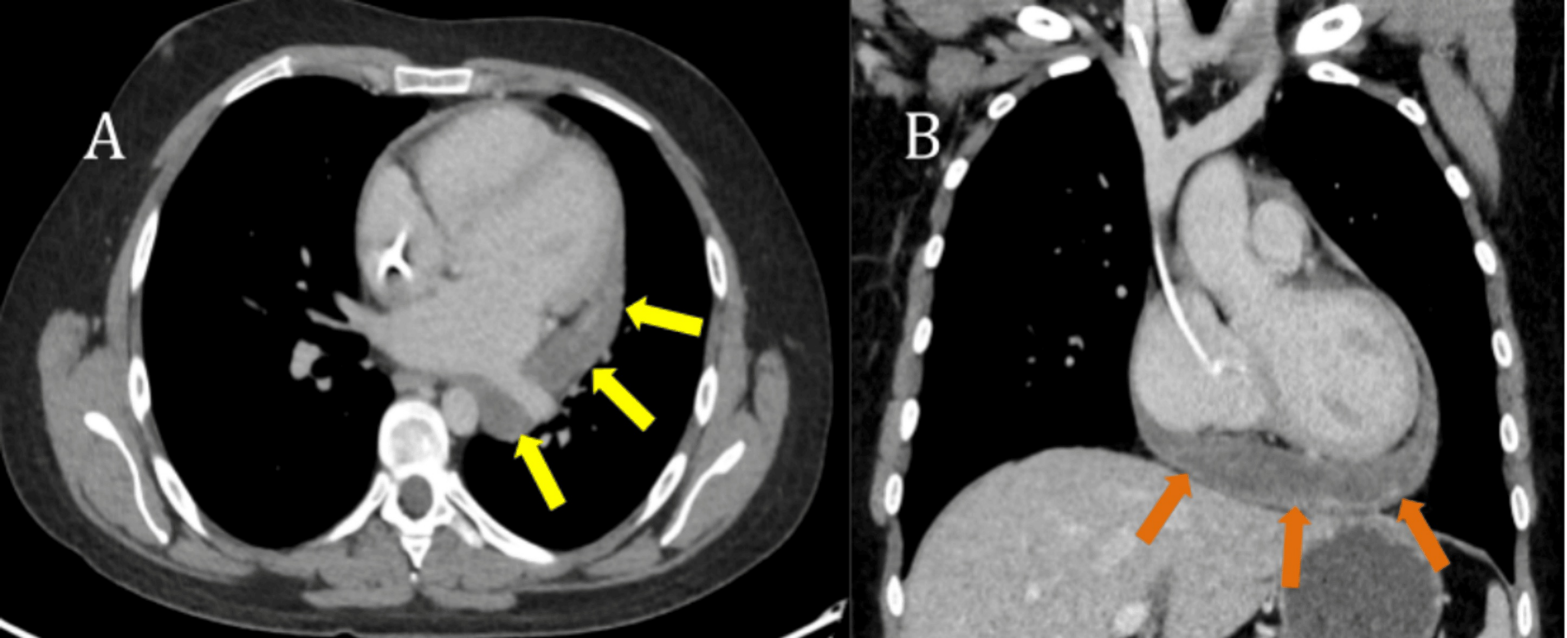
(A) Axial CECT of the chest with a decrease in the size of the pericardial mass (yellow arrows). (B) Coronal CECT of the chest with a decrease in the size of the pericardial mass (orange arrows). CECT: contrast-enhanced computed tomography
